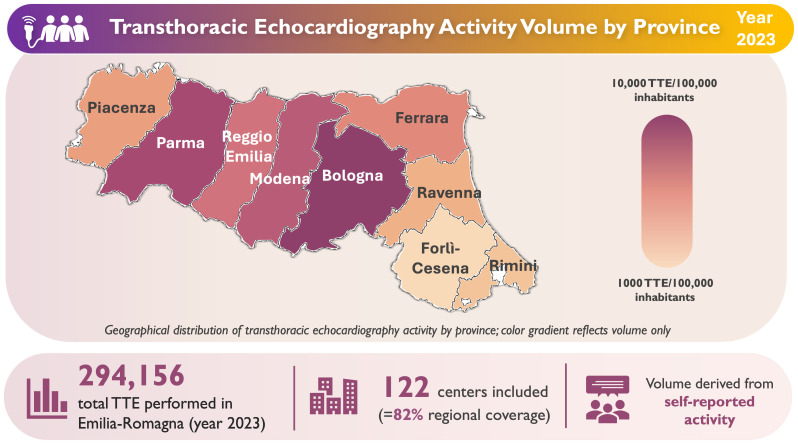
Transthoracic echocardiography (TTE) activity by province in Emilia-Romagna (2023). Annual TTE volume per 100,000 inhabitants, estimated from self-reported monthly activity (×12) and standardized to provincial population data (ISTAT 2023).
